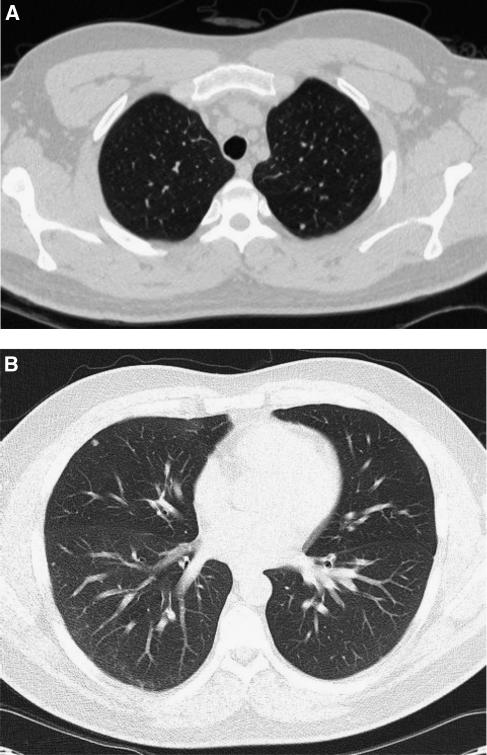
(A) lung nodule posteriorly left upper lobe; presumed granuloma, unchanged on sequential chest CT. (B) lung nodules anteriorly right middle lobe and laterally right lower lobe; presumed granuloma, unchanged on sequential chest CT.
